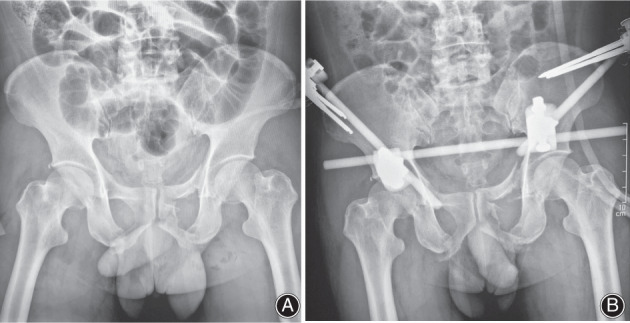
A 50‐year old male patients with HE‐PFs. (A) Pelvic radiograph showed superior and inferior ramus of pubis fracture. (B) Another pelvic radiography of the same patient was taken 1 week after closed reduction and external fixation
